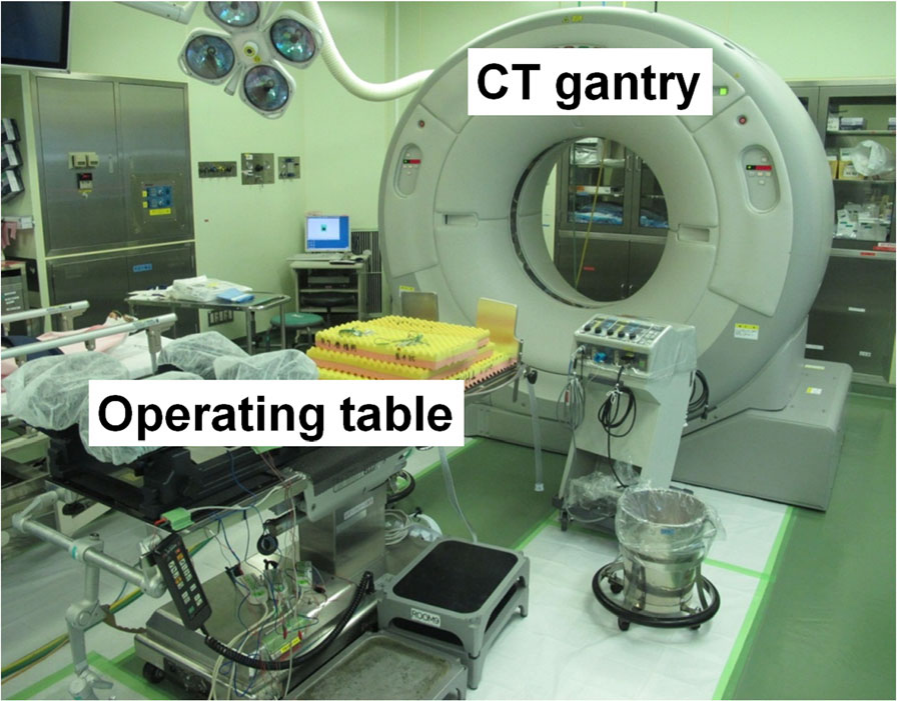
A multidetector CT with mobile gantry in the operating room
